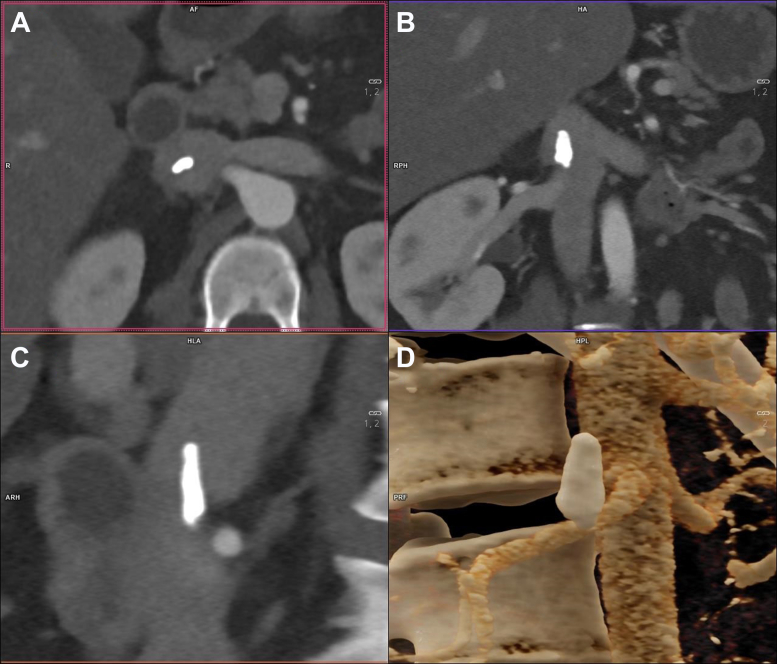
Computed tomograhy (CT) scans of bullet thrombus within the inferior vena cava (IVC) located below the right renal vein (axial [A], coronal [B], and sagittal [C] planes and corresponding three-dimensional reconstruction [D]).
